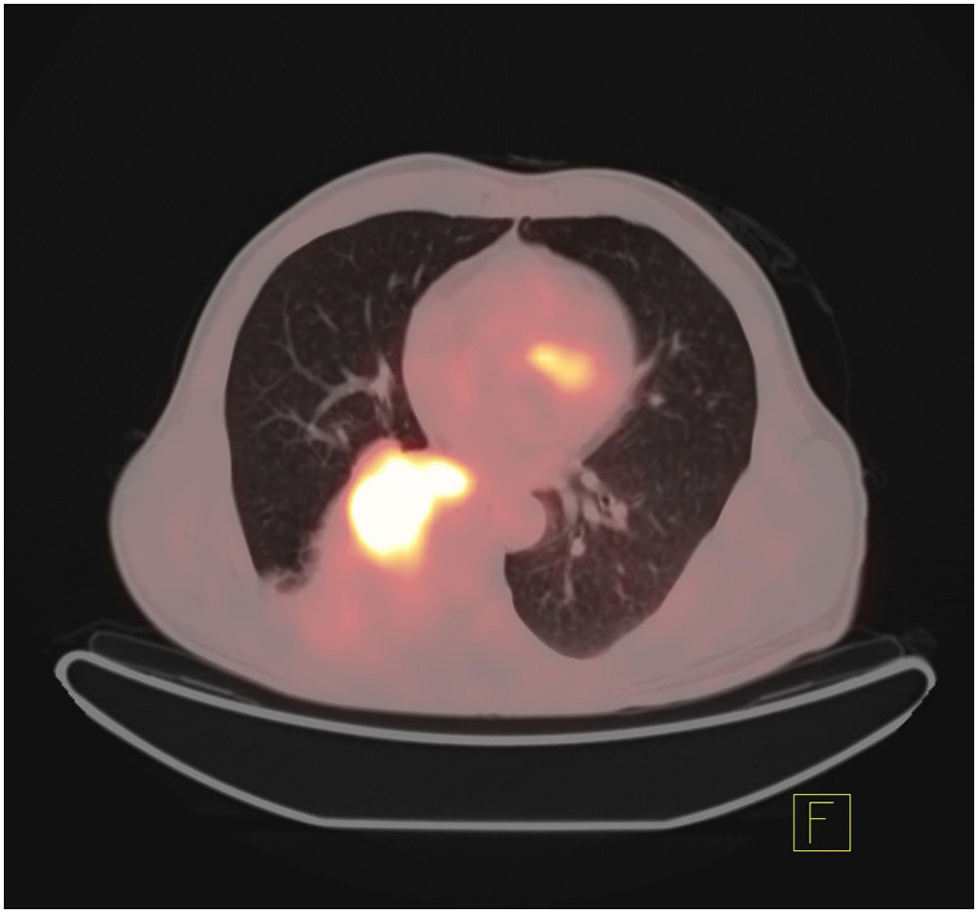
PET/CT manifestations of EGFR wild-type patients with lung adenocarcinoma.
